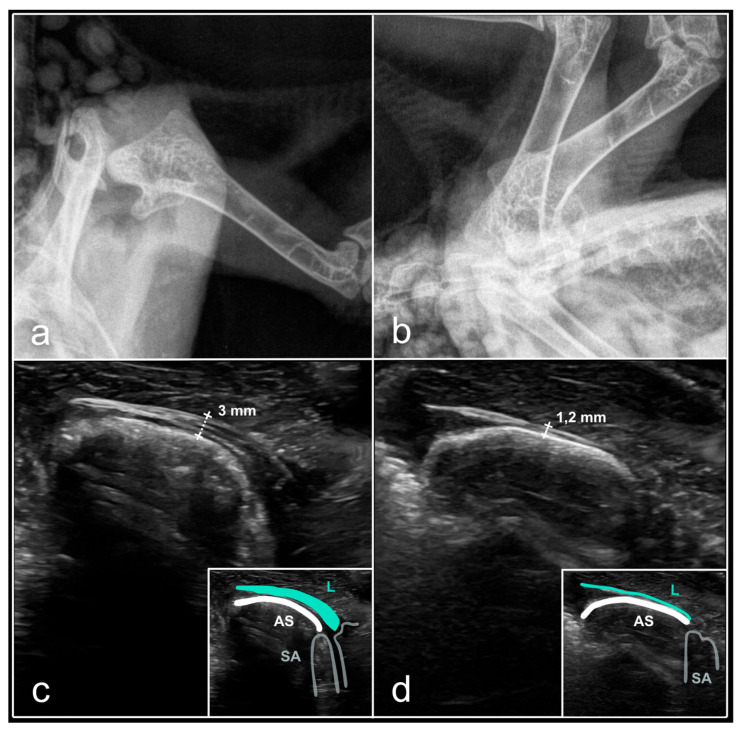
Tendinitis of the shoulder joint (*Articulatio humeri*) in the feral pigeon (*Columba livia* f. domestica). (a)—ventrodorsal X-ray, pathology not evident; (b)—laterolateral X-ray, pathology not evident; (c)—B-mode ultrasound, longitudinal section, craniodorsal access, the joint surface of the humeral head (*Caput humeri*) appears irregular, the acrocoracohumeral tendon (*Ligamentum acrocoracohumeralis*) exhibits a ‘beak-shaped’ morphology and is markedly thickened (3 mm in the central region) ‘hockey stick’ transducer, 15 MHz; (d)—B-mode ultrasound image of the intact shoulder joint, longitudinal section, craniodorsal access, the joint surface of the humeral head (*Caput humeri*) appears as a hyperechoic flat structure, the acrocoracohumeral tendon measures 1.2 mm in thickness in the central region, ‘hockey stick’ transducer, 15 MHz. Legends: AS—articular surface of the humeral head (*C. humeri*), L—acrocoracohumeral tendon (*L. acrocoracohumeralis*), SA—shadow artefact (acoustic artefact). Source: A. Korshunova.
